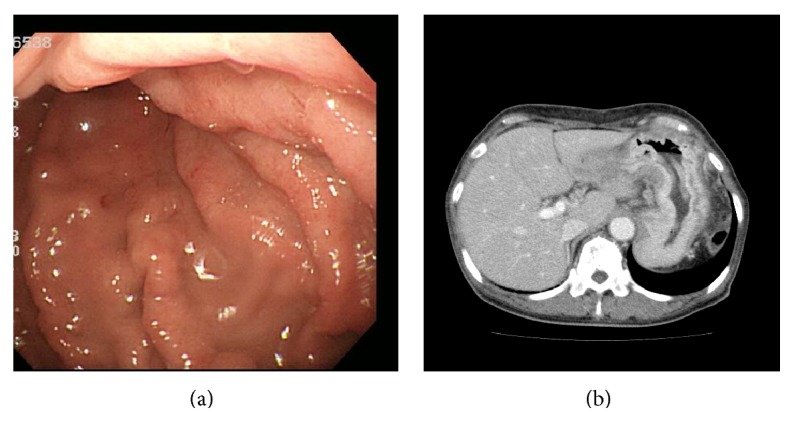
(a) Upper endoscopy. Mucosal rugae were edematous and thickened. (b) Contrast-enhanced CT revealed diffuse thickening of gastric wall.
